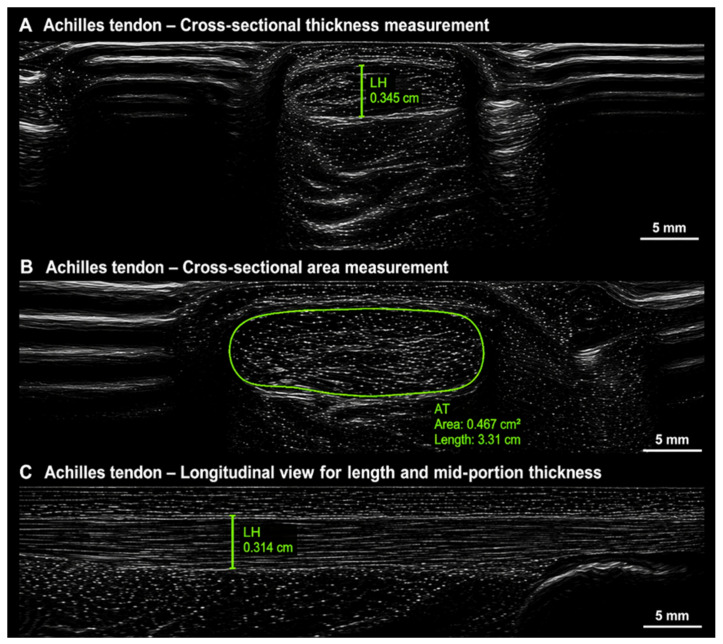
Representative ultrasound images of the Achilles tendon. (A) Cross-section thickness measurement at the superficial, midpoint, and deep regions. (B) Cross-sectional area measurement of the Achilles tendon. (C) Longitudinal view demonstrating tendon thickness at the mid-portion. Scale bars = 5 mm. Abbreviations: LH = lateral thickness; AT = Achilles tendon; cm = centimeters; cm^2^ = square centimeters.
